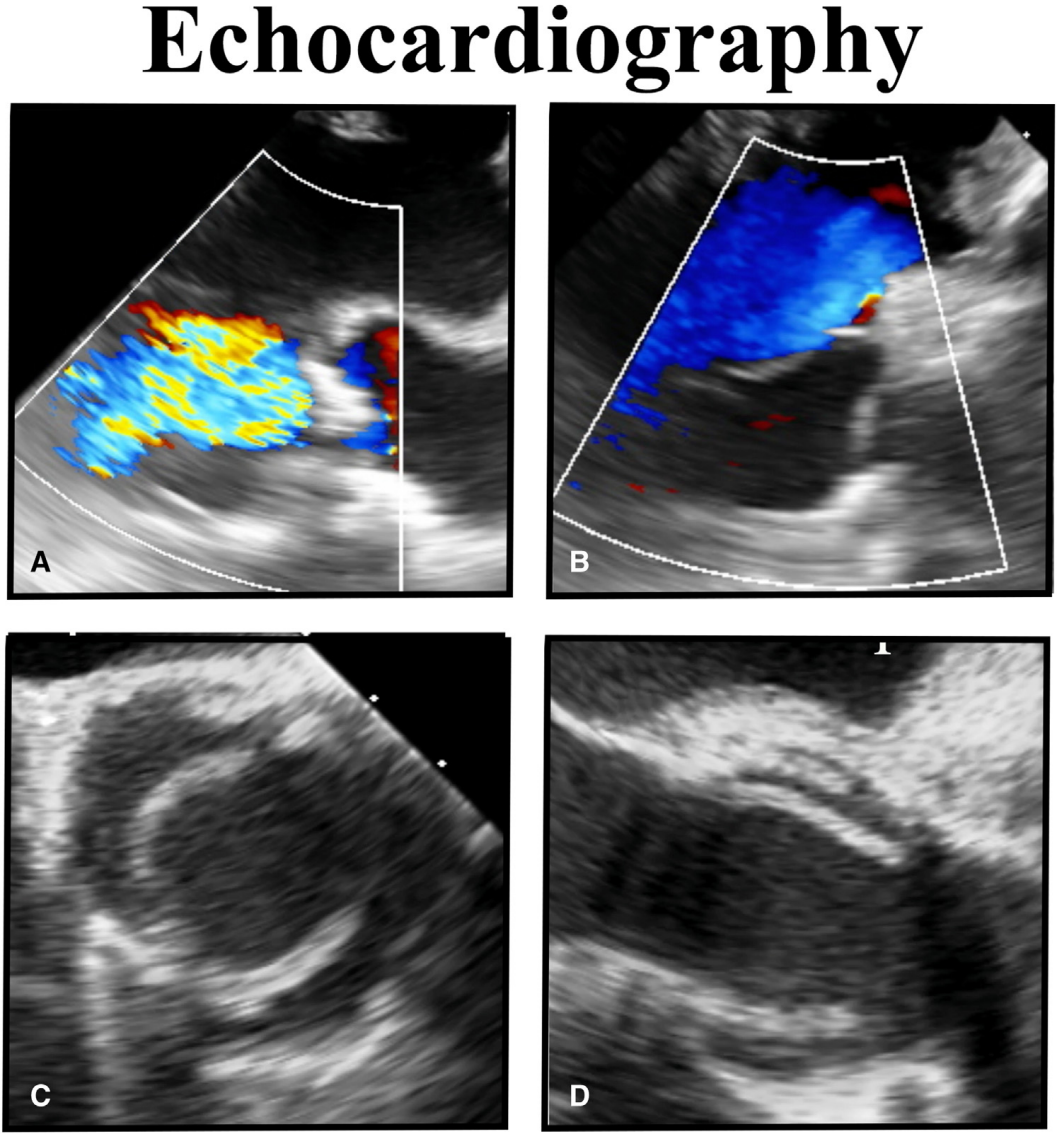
Echocardiography of autologous tissue valve. A, Calcified leaflets are moderately severely insufficient preoperatively. B, After repair, the autologous valve is fully competent. C, Repaired valve opens well during systole. D, Repaired aortic wall leaflets are tall and parallel during ejection.
